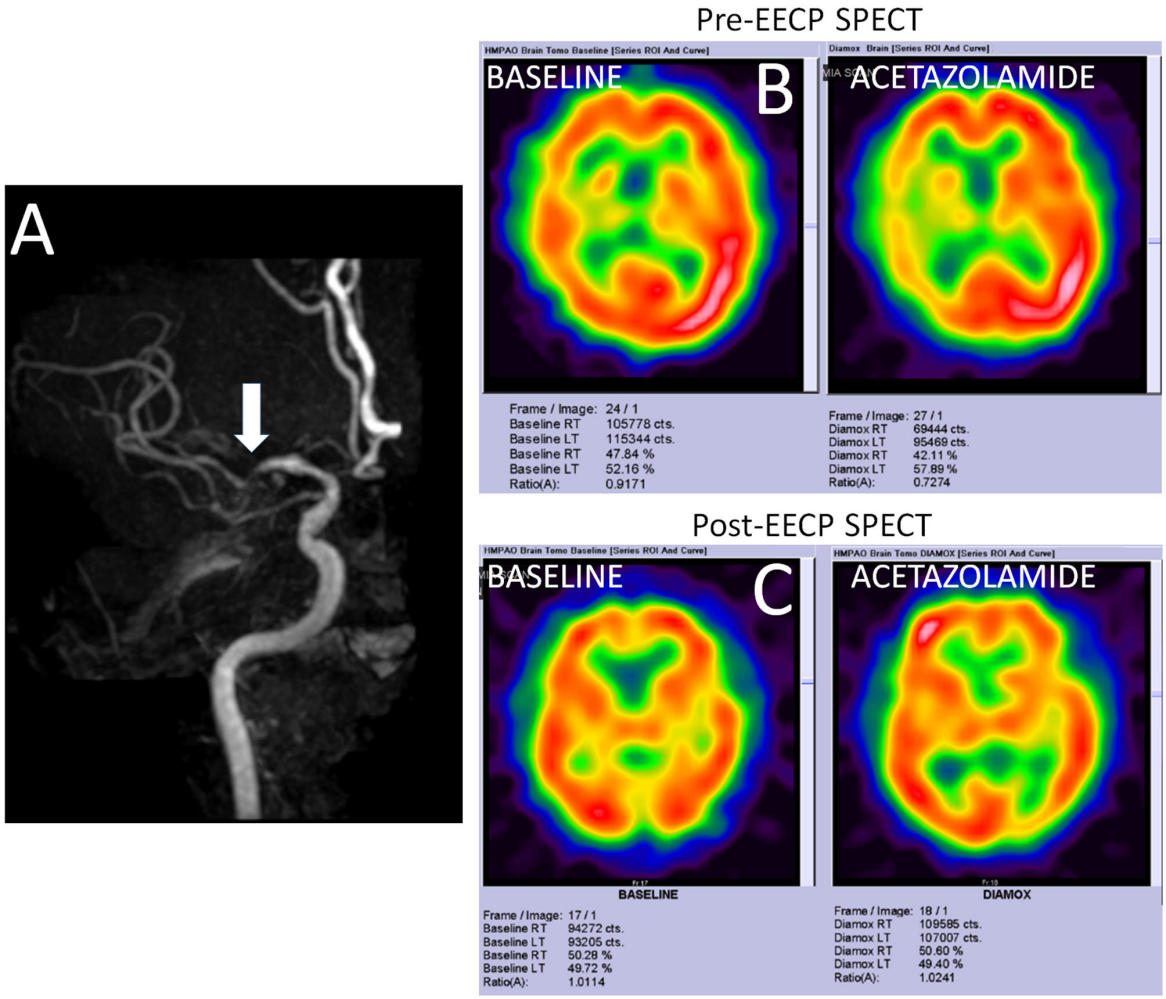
Imaging findings in a study participant. (A) Shows the time-of-flight MR angiography in a patient with severe stenosis of right middle cerebral artery (MCA). (B) Shows the SPECT findings at the baseline and after acetazolamide challenge. While only mildly reduced metabolic perfusion is noted in the right MCA territory at rest, acetazolamide challenge reveals a significantly impaired vasodilatory reserve (net perfusion deficit 11.46%). (C) Shows normalization of the metabolic perfusion at the baseline as well as after acetazolamide challenged SPECT.
